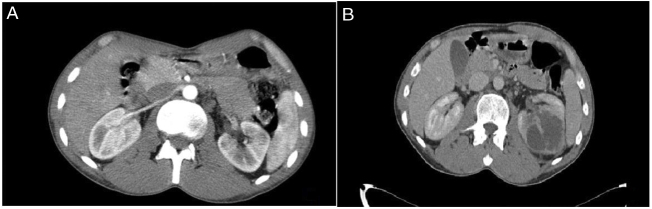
(A) Abdominal CT scan in at the time of diagnosis, (B) Abdominal CT scan axial view of left renal mass. A solid expansive lesion of the medial pole of the left kidney, with the presence of bilobed hypodense area of cystic appearance approximately 40 × 24 mm in the renal parenchyma.
